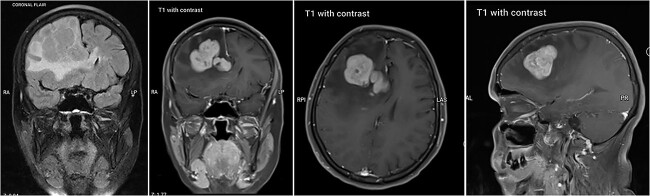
Brain MRI showing right-sided fronto-parietal mass.
